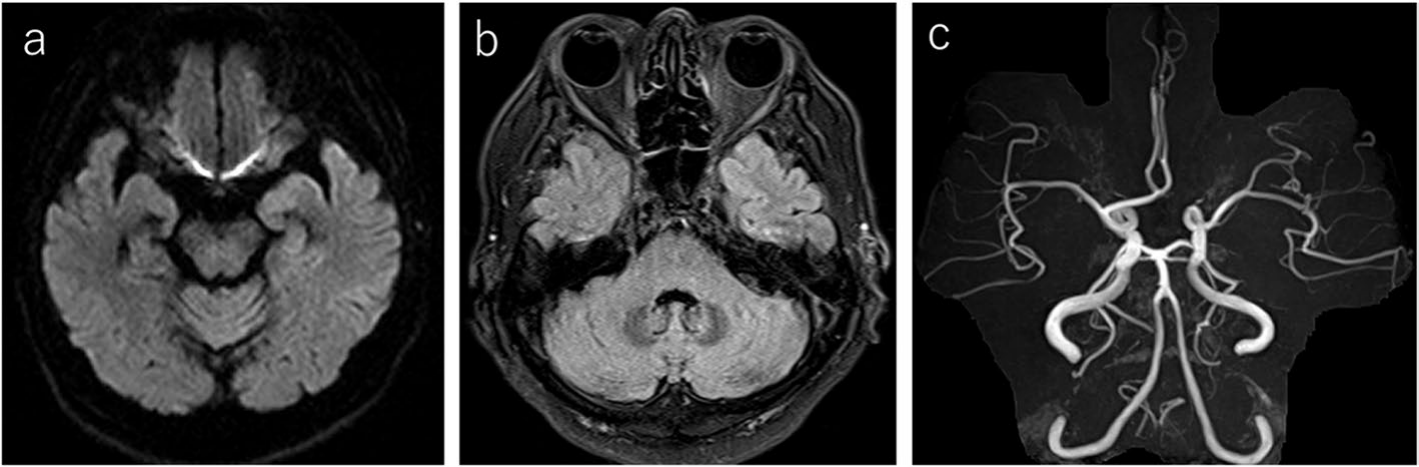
a Diffusion-weighted magnetic resonance images, b Fluid-attenuated inversion-recovery images, and c Magnetic resonance angiography shows no abnormalities
